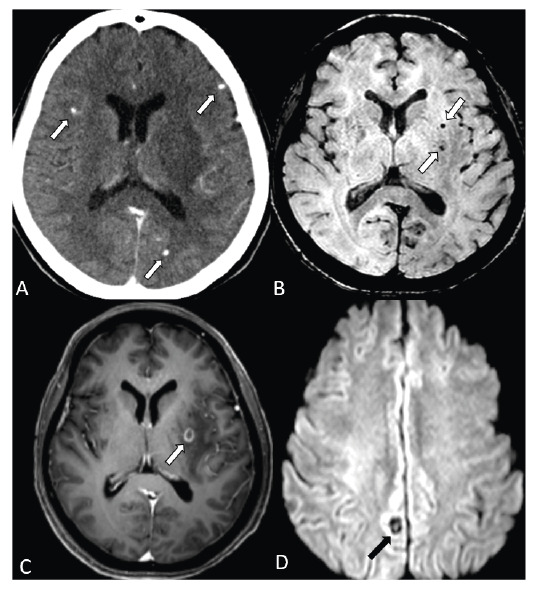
(A) Axial computed tomography reveals multiple sub-centimeter calcified lesions (arrows) predominantly throughout the supratentorial brain. (B) Axial susceptibility-weighted imaging (SWI) demonstrates multiple round hypointense foci, consistent with calcifications (arrows). (C) Axial contrast-enhanced T1-weighted image (WI) reveals a cystic ring-enhancing lesion (arrow) with perilesional edema in the left putamen. (D) Axial diffusion-weighed imaging (b=1000) reveals a hyperintense eccentric dot (scolex, arrow) indicative of neurocysticercosis in the colloidal vesicular stage.
